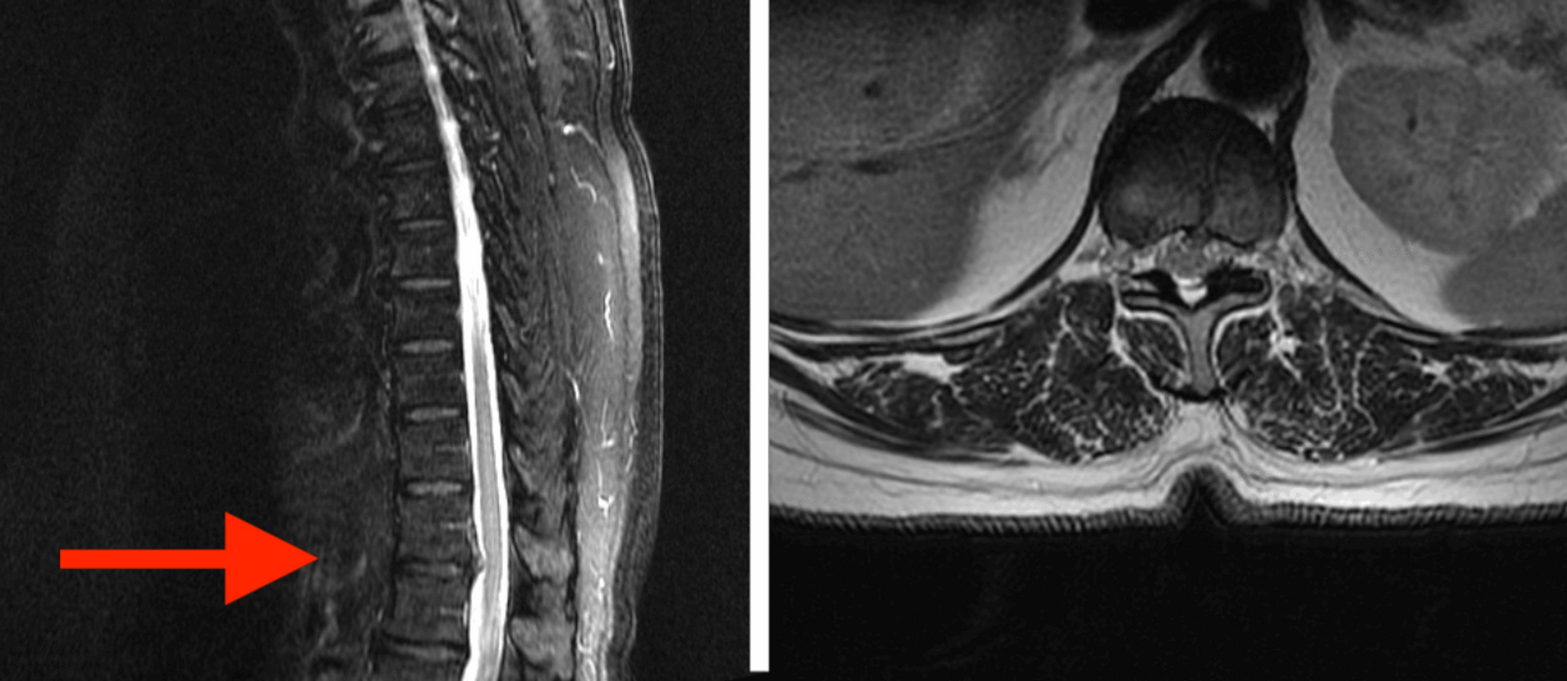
Preoperative imaging demonstrating thoracic disc herniation Preoperative magnetic resonance imaging (MRI) in the sagittal (A) and axial (B) planes revealing disc extrusion at T11-T12 combined with ligamentum flavum infolding causing mild central canal narrowing and resulting in a mass effect on the cord. The arrow in (A) indicates the T11-T12 level.
